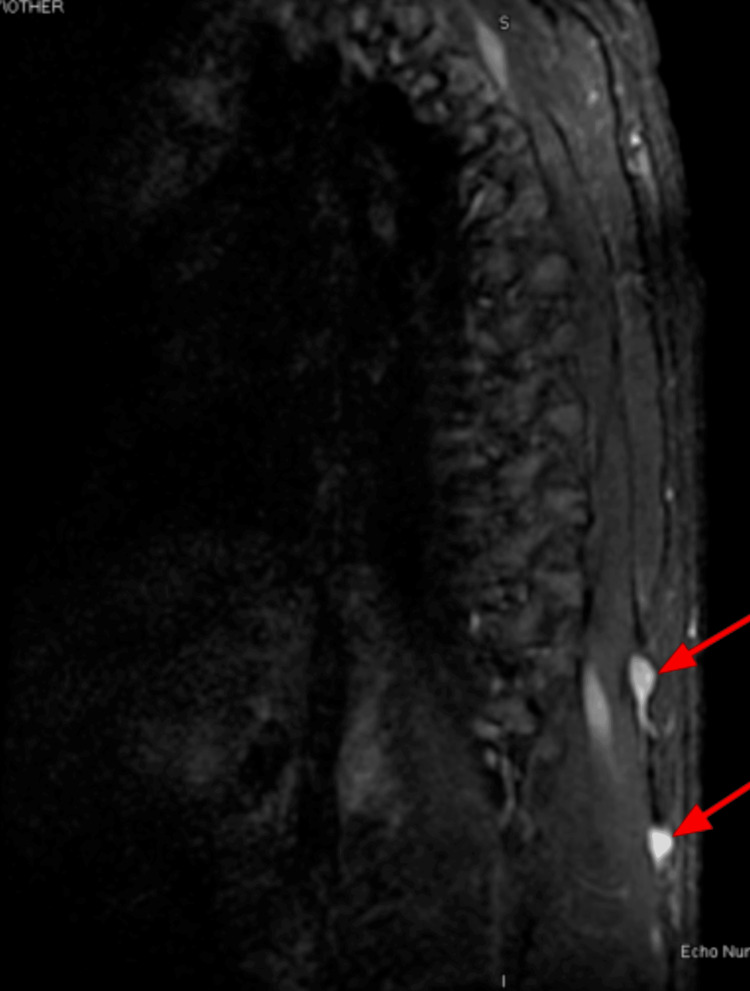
Spinal MRI (April 12, 2023) showing multiple enhancing hemorrhagic lesions in the soft tissue
